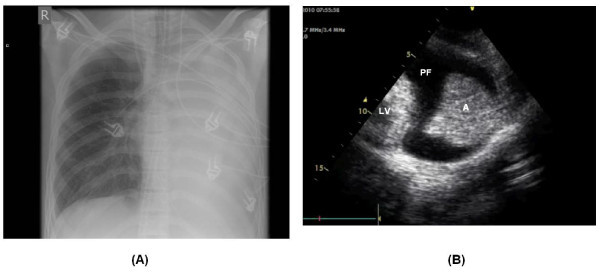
Chest X-ray and ultrasound of patient 2. (A) Chest X-ray showing total opacification of the left lung. (B) Lung ultrasound with atelectasis of the left lung (A) and hypoechogenic area of pleural effusion (PF).
